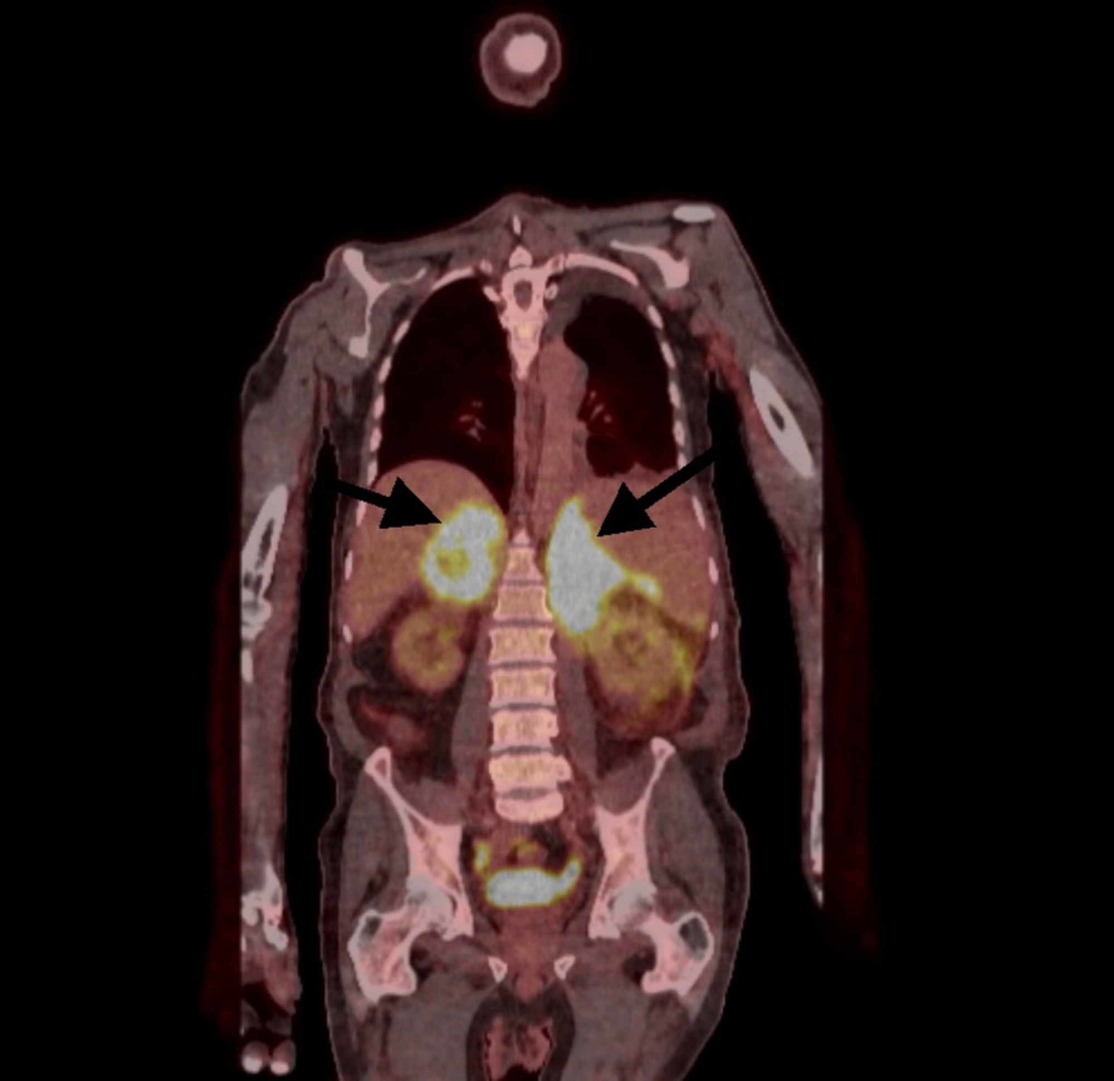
Positron emission tomography/computed tomography (PET/CT) coronal view Intensely hypermetabolic disease within the abdomen, involving both the adrenal glands, with conglomerate 18F-fluorodeoxyglucose (FDG) avid soft tissue extending inferiorly from the retroperitoneum and mesentery predominantly along the left side, extending for at least 21 cm craniocaudal standardized uptake value (SUV) max 15.4.
